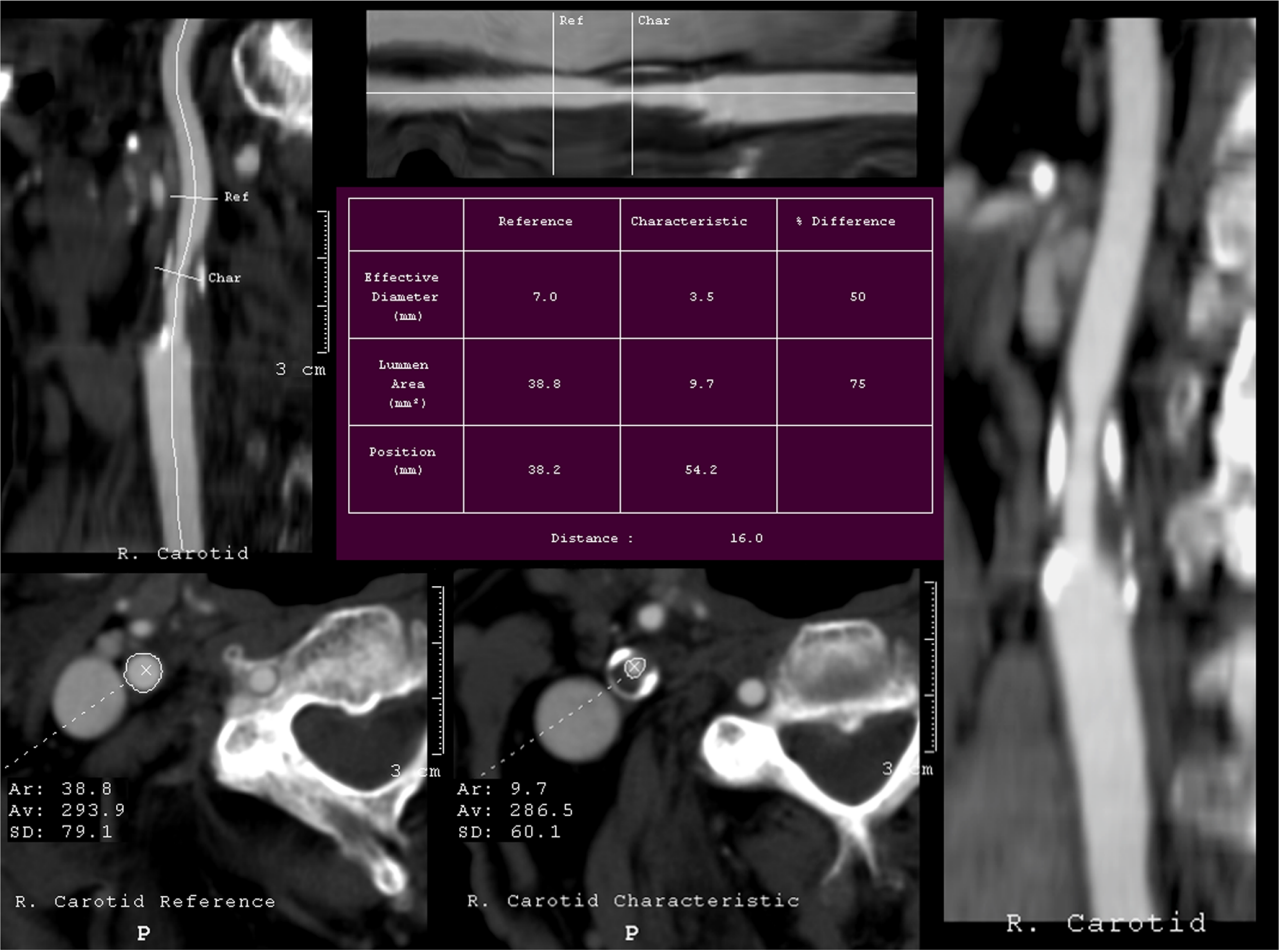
Automated quantification of an eccentric right carotid artery stenosis on CTA. Percentage of stenosis is calculated by electronically outlining the residual and the normal vessel lumen in a plane perpendicular to the longitudinal axis of the vessel. In this example, the area of the residual lumen is 9.7 mm^2^, the area of the normal lumen is 38.8 mm^2^, resulting in 75% stenosis. The residual lumen diameter is 3.5 mm, the normal lumen diameter is 7.0 mm, resulting in 50% stenosis
